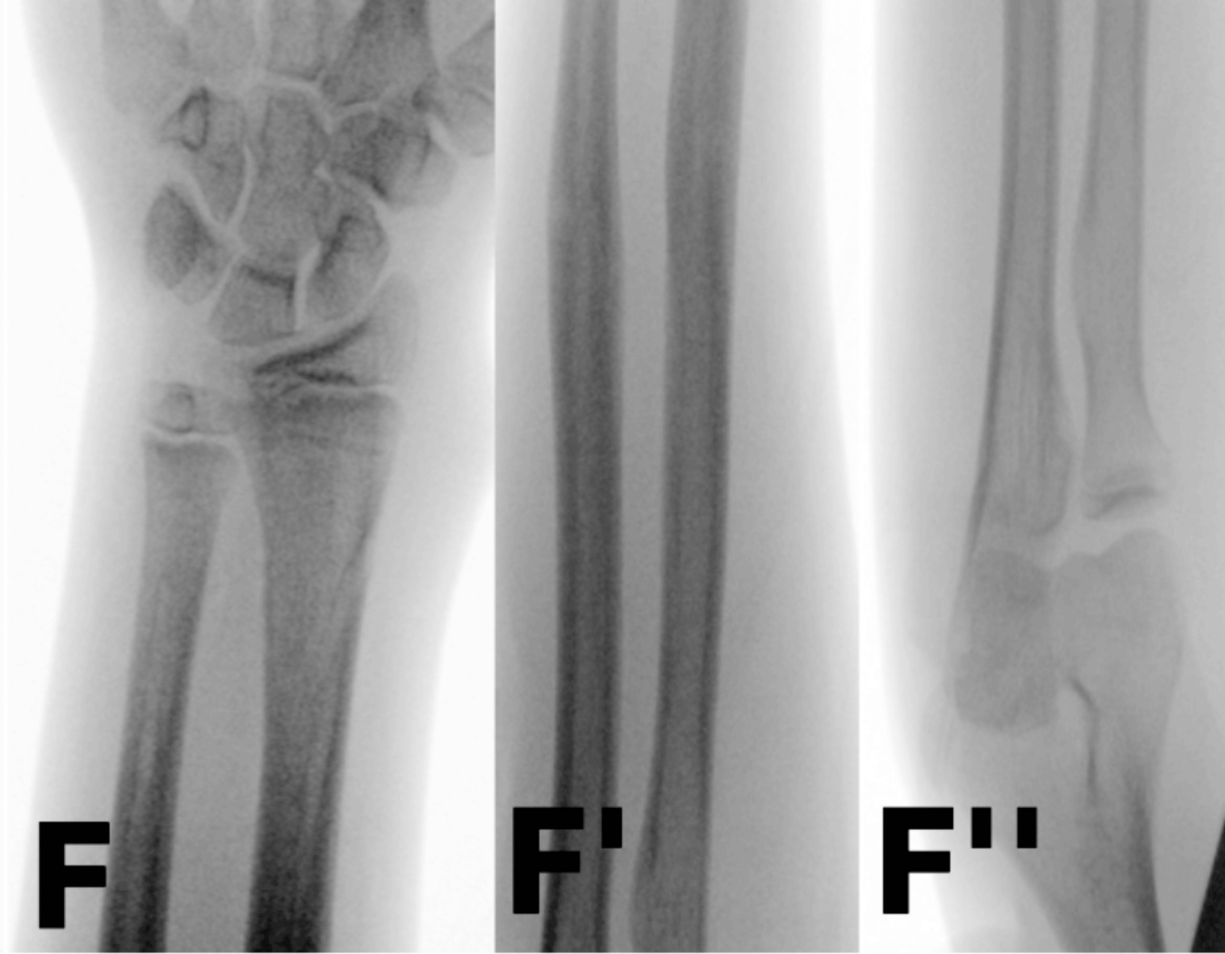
Intraoperative radiographs showing uncomplicated removal of TENS AP view of the wrist (F), forearm shaft (F'), and proximal forearm (F'') during TENS removal TENS: Titanium elastic nailing system
